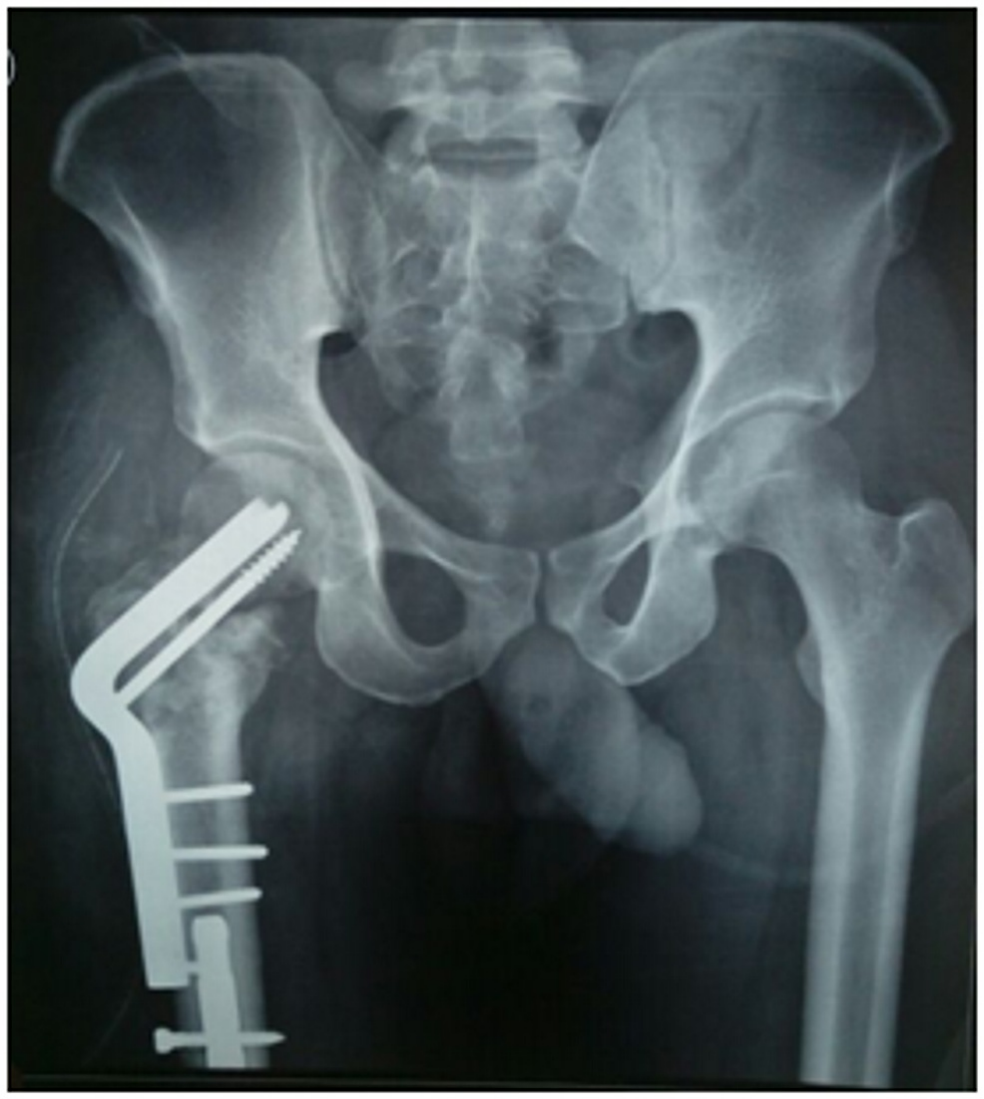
Immediate postoperative radiograph showing fixation of the Pauwels' intertrochanteric osteotomy with angled blade plate
